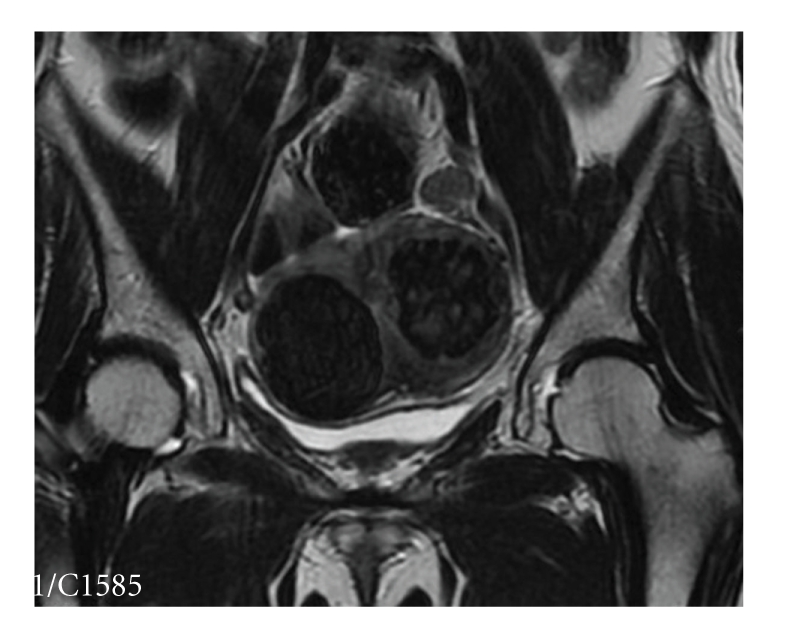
Coronal T2-weighted image three months post-treatment, showing 49% volume shrinkage of the treated fibroid.
